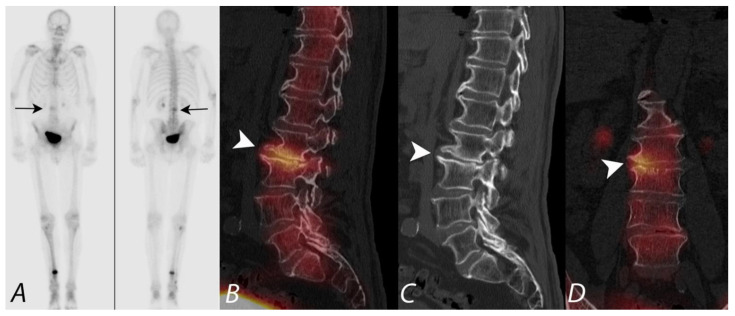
Anterior and posterior planar images (A) from a ^99m^Tc MDP bone scan on a patient with prostate cancer being evaluated for metastatic disease, with focal uptake along the right aspect of lumbar spine (black arrows). Additional focus of uptake involving the distal right tibia is related to a known fracture involving this region. No additional foci of uptake concerning metastatic disease were identified. Sagittal CT image and fused SPECT/CT images in sagittal and coronal projections (B–D) localize the activity to the right aspect of the disc space between L2 and L3, correlating with disc space narrowing and osteophytosis (white arrowhead). Findings are consistent with uptake related to degenerative disc disease and not related to metastatic disease.
